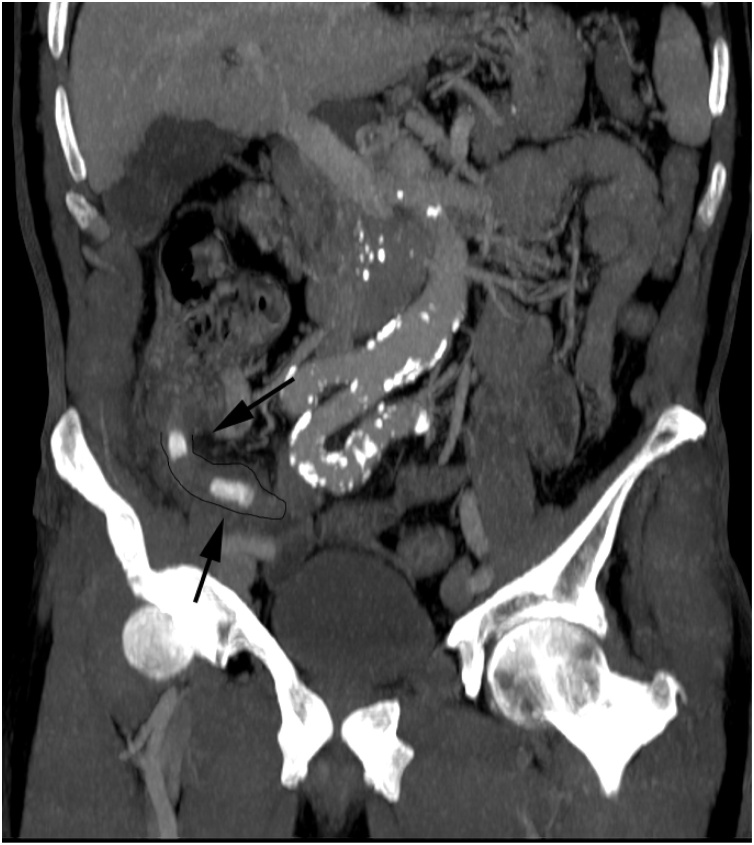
CT scan showing perforated appendix containing two appendicoliths.
